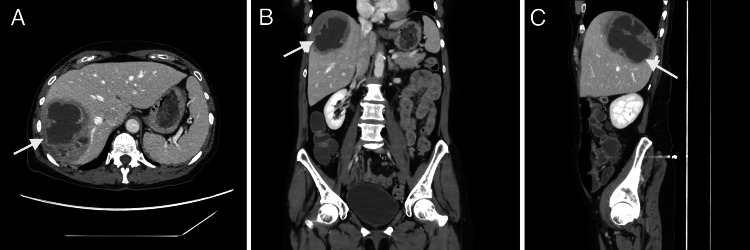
Contrast-Enhanced Computed Tomography (CT) of The Abdomen (A) Coronal (B) and sagittal (C) views of a lobulated, peripherally enhancing fluid collection within the right hepatic lobe, involving segments 5, 6, 7, and 8 (white arrow). The lesion measures approximately 6.1 x 8.1 x 8.9 cm and is surrounded by adjacent parenchymal edema, consistent with a hepatic abscess.
